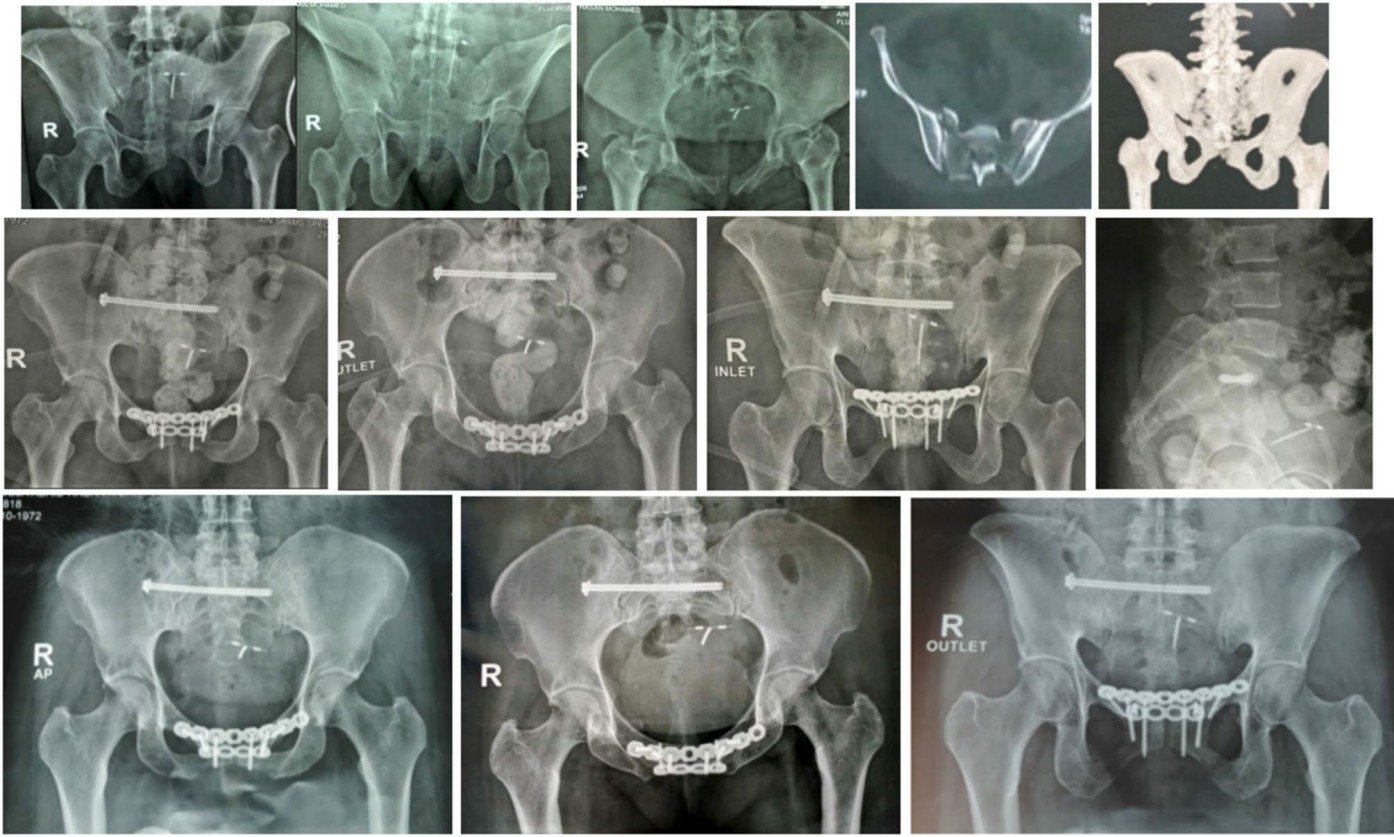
A 48-year-old female fell from a height. a-e preoperative radiographs and computed tomography (CT) scan showing Tile C1-3 injury (VS Fracture Sacrum); f-i postoperative radiographs showing posterior ring fixation with SI screw and anterior ring fixation via double superior and anterior symphyseal plates. J-L radiographs taken 15 months postoperatively
